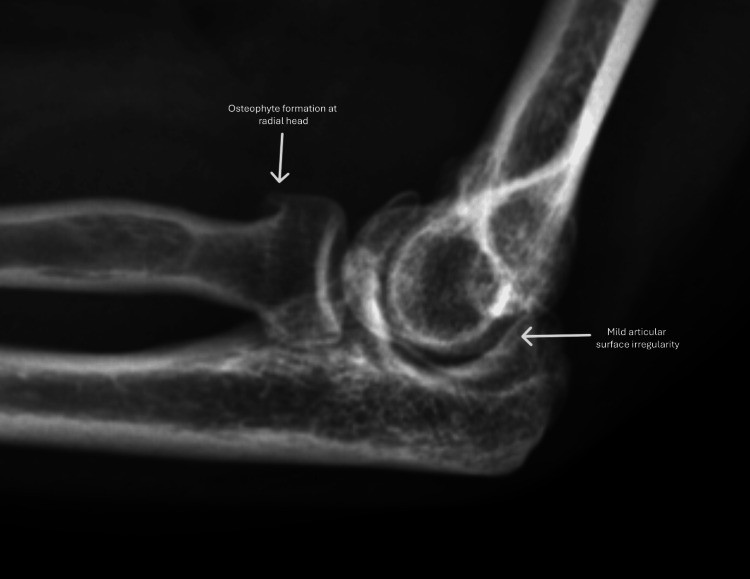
Lateral radiograph of the left elbow demonstrating early degenerative and neuropathic changes, including radial head osteophyte formation and mild articular surface irregularity, suggestive of early ipsilateral joint involvement.
